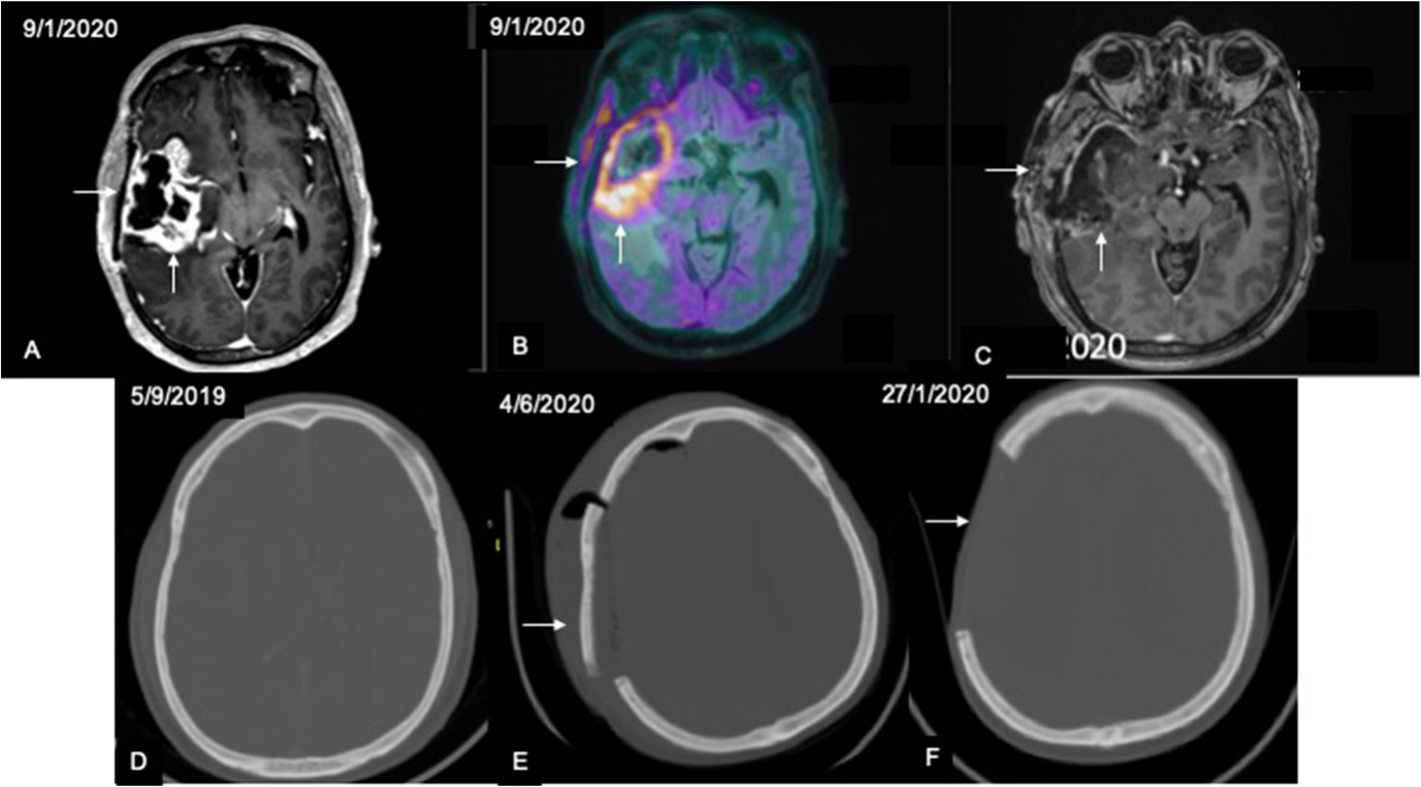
Sequence of images showing false positive C11 methionine uptake in acute inflammatory pathology: Axial post-contrast T1 MPRAGE (A) in a case of suspected recurrence shows parenchymal enhancement (vertical arrow) suggestive of recurrent lesion with associated enhancement of the overlying bone and soft tissues (horizontal arrow). C11 methionine PET image fused with axial T1 MPRAGE (B) shows tracer uptake along the bone and soft tissues (horizontal arrow) in addition to parenchymal uptake (vertical arrow). Here the uptake in the bone and soft tissues occurred due to osteomyelitis. Follow-up axial post-contrast T1 MPRAGE following chemo/radiotherapy (C) shows reduction in parenchymal enhancement (vertical arrow) with persistent enhancement in bone and soft tissues (horizontal arrow). Sequential axial CT brain images in bone window (D–F) show initial scan (D) with normal bone followed by progressive osteomyelitis in E and excision of the infected bone in F
